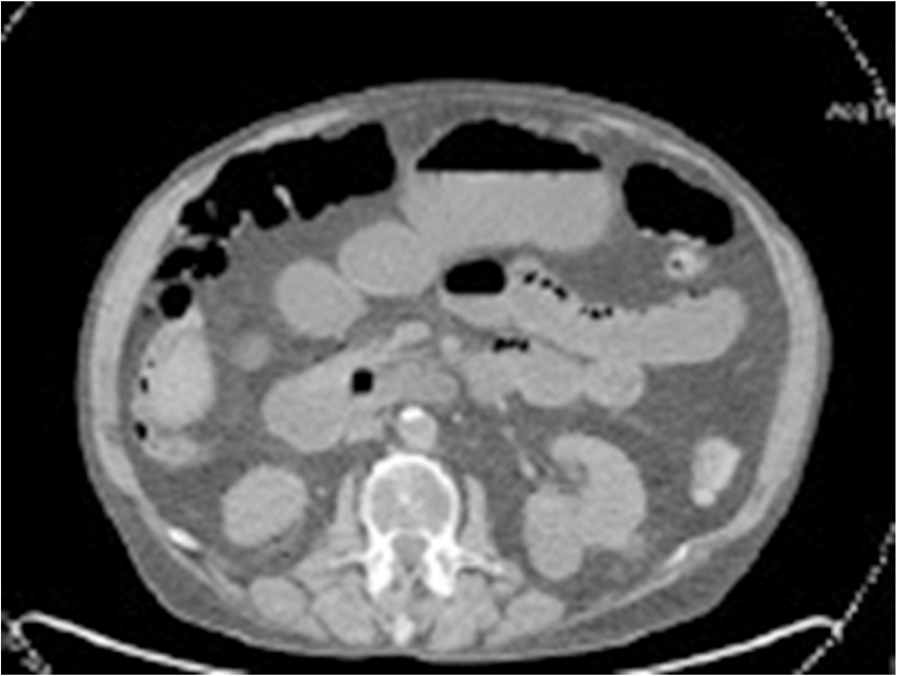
CT scan of the abdomen. CT scan of the abdomen showing a distention of small bowel loops with several air-fluid levels.
